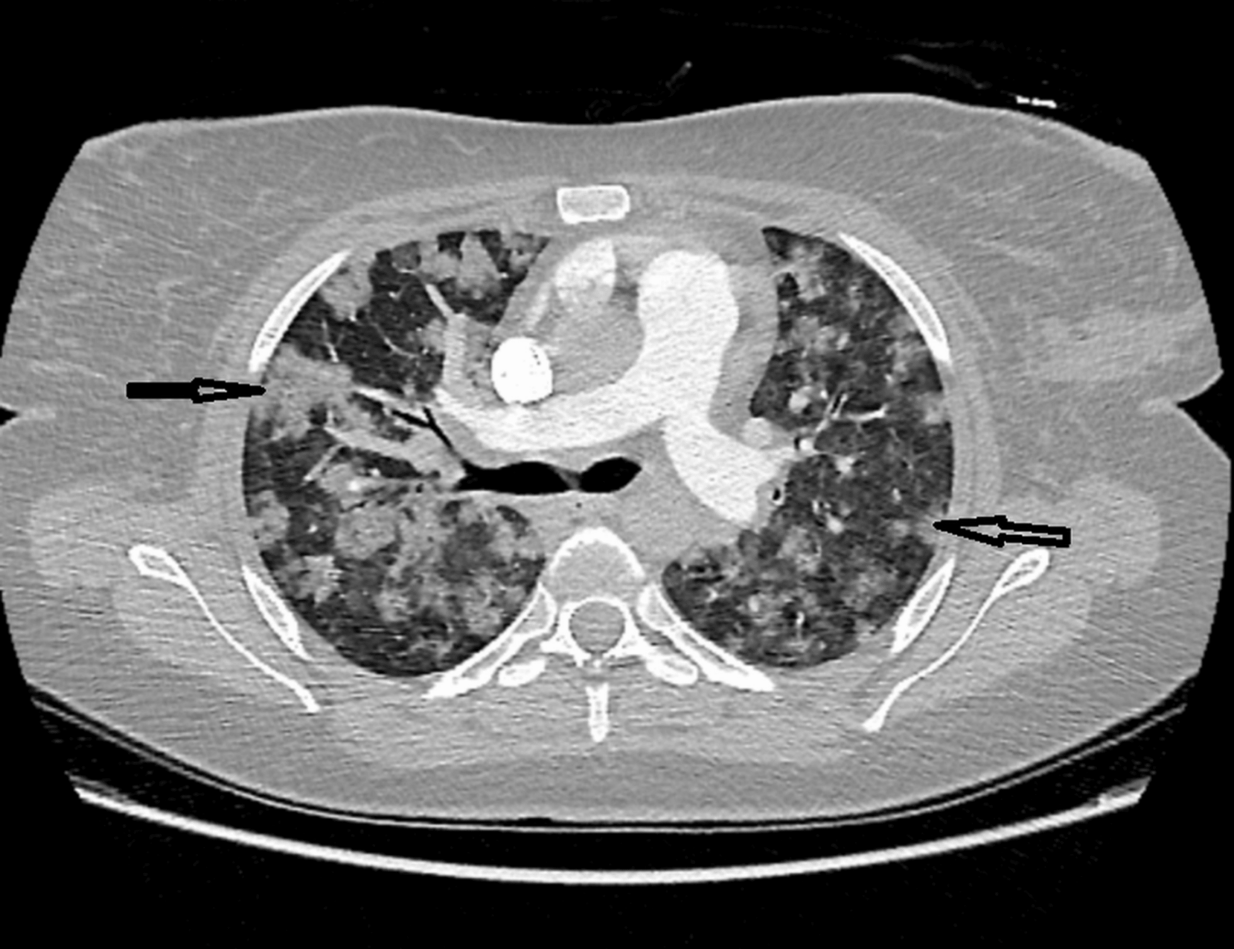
Computed tomography of the chest Axial contrast-enhanced computed tomography scan of the chest demonstrating bilateral diffuse ground-glass opacities with a crazy-paving pattern (arrows), consistent with diffuse alveolar hemorrhage.
